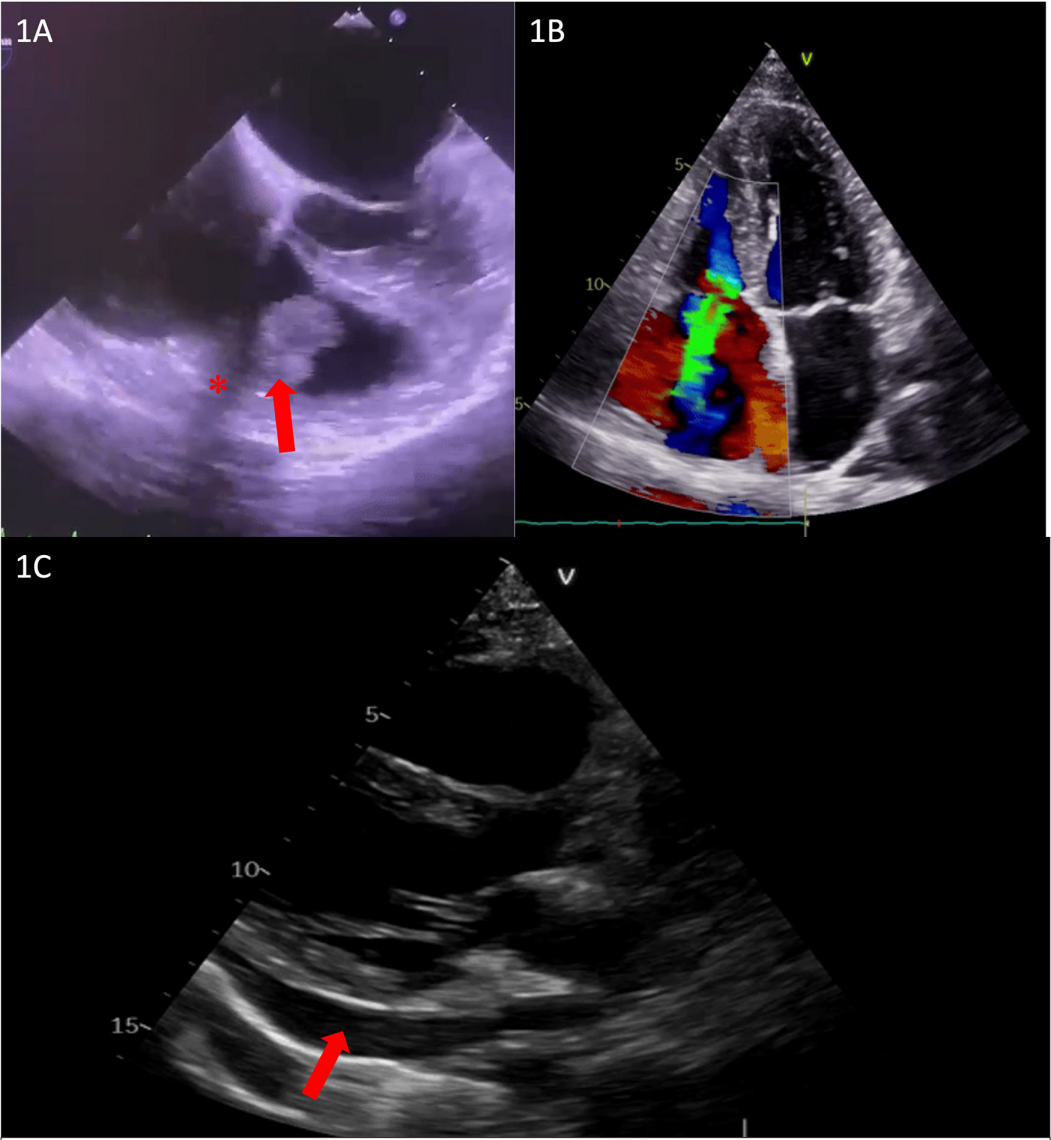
(A) Transesophageal echocardiography showing subvalvular vegetation (red arrow) and unusual echocardiographic shadowing (red asterisk). (B) TTE showing severe tricuspid regurgitation. (C) Transthoracic echocardiography showing pericardial effusion up to 14 mm (red arrow).
